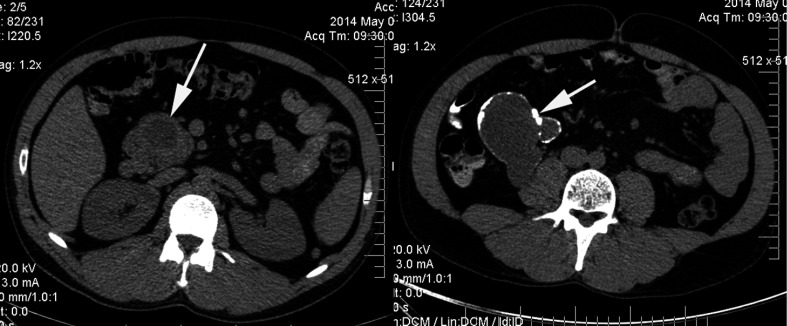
Preoperative CT scan. A cyst originated from the uncinate process of pancreas (indicated by arrow) is shown on the left slice. The right slice is taken from a lower part of the abdomen. Arrow indicates a multivesicular cyst with wall calcification
